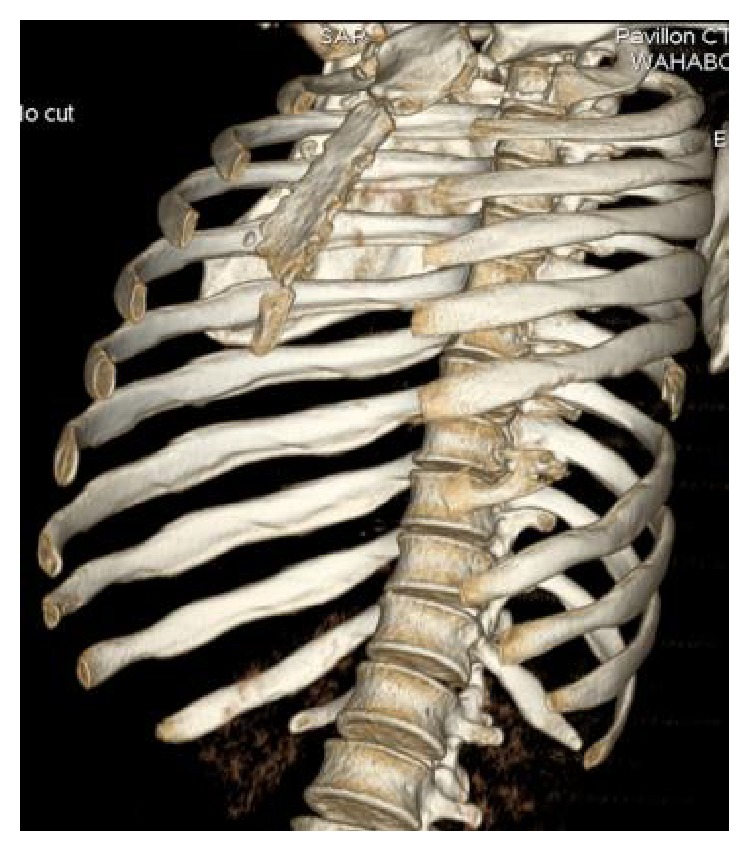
Computed tomography 3D reformation showing destruction of the 7th rib.
